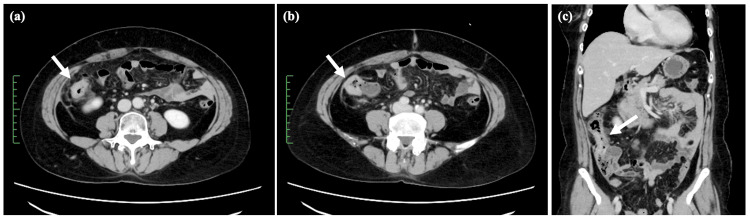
Abdominal CT images after anastrozole and palbociclib therapy. A metastatic lesion in the ascending colon (indicated by the arrow) shows no obvious tumor enlargement (a, b, c).
